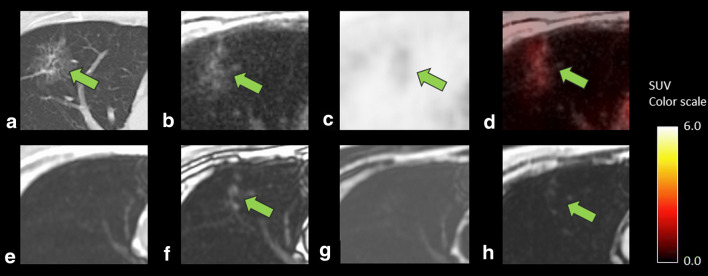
A case showing lung adenocarcinoma in a 61-year-old man after surgery for pharyngeal cancer. CT (a) showed a 7.3-mm-sized lesion with ground-glass opacity component. ZTE (b) can reveal these CT characteristics, leading to correct diagnosis of lung cancer by the combined findings of the FDG uptake on PET (SUVmax 3.9, c) and fused images (d). Dixon sequence (e, in phase; f, out of phase; g, fat; and h, water image, respectively), however, failed to depict the characteristics of ground-glass opacity, resulting in the diagnosis of differentiation as equivocal
